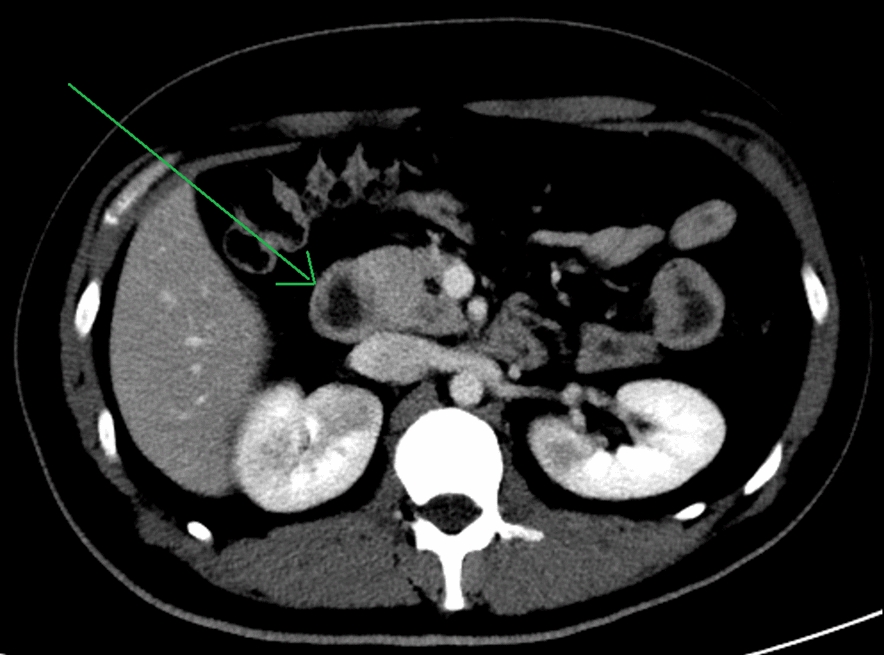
Axial view of abdominal computed tomography angiograph, with the arrow pointing to a 7.1 mm thickening of the second duodenal segment, suggesting duodenitis
